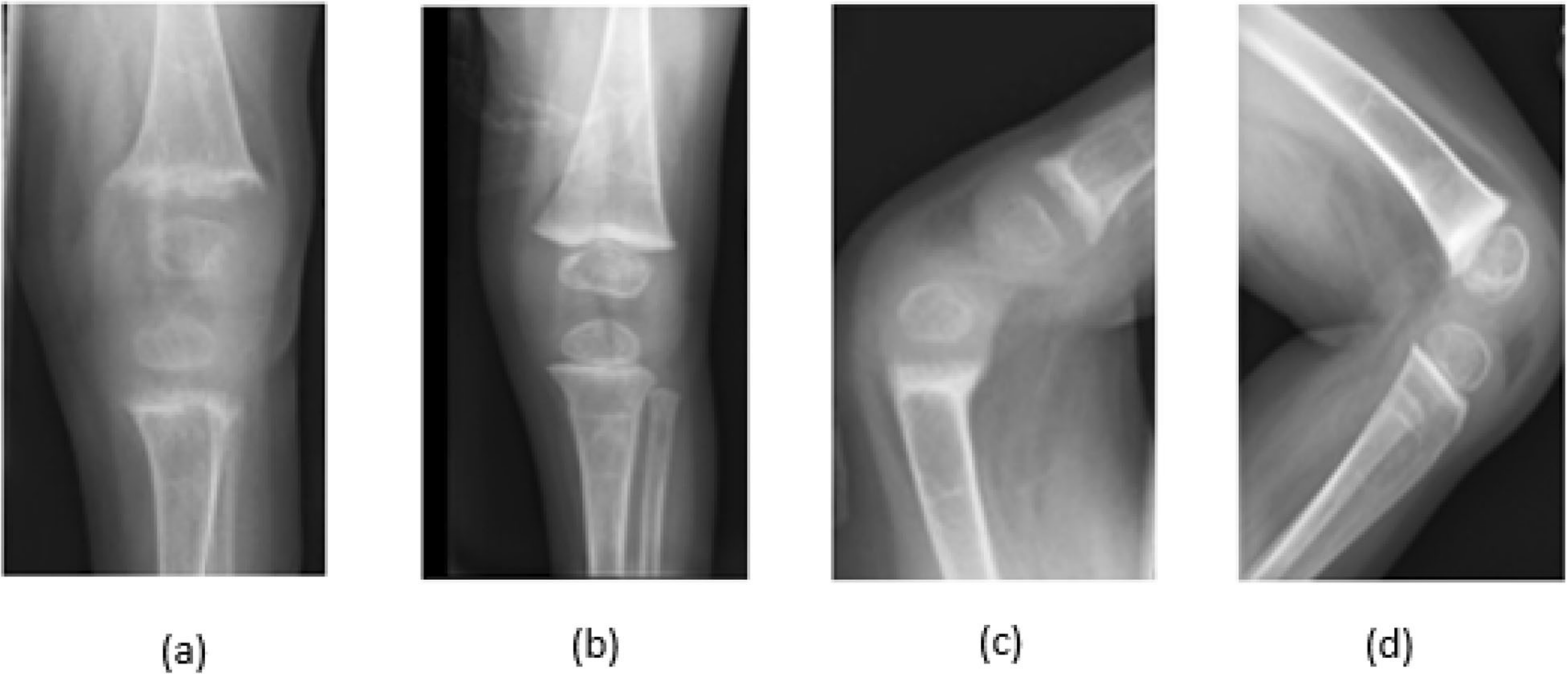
Twin* A knee radiographs. AP view (a) before treatment (b) after treatment. Lateral view (c) before treatment and (d) after treatment. a and c show diffuse osteopenia, fraying and widening of metaphyses. b and d demonstrate improvement in rickets
